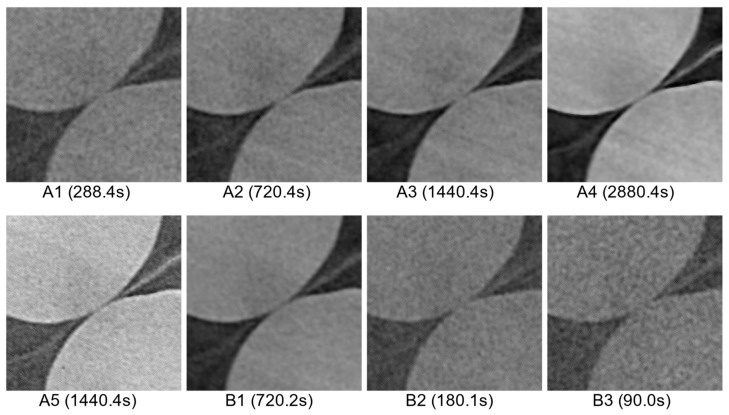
Section of horizontal XCT slices depicting two non-contacting glass pellets separated by a layer of PE film (seen as a diagonal streak) for different acquisition set-ups (Table 1). Total X-ray exposure time for each scan is shown in parenthesis.
